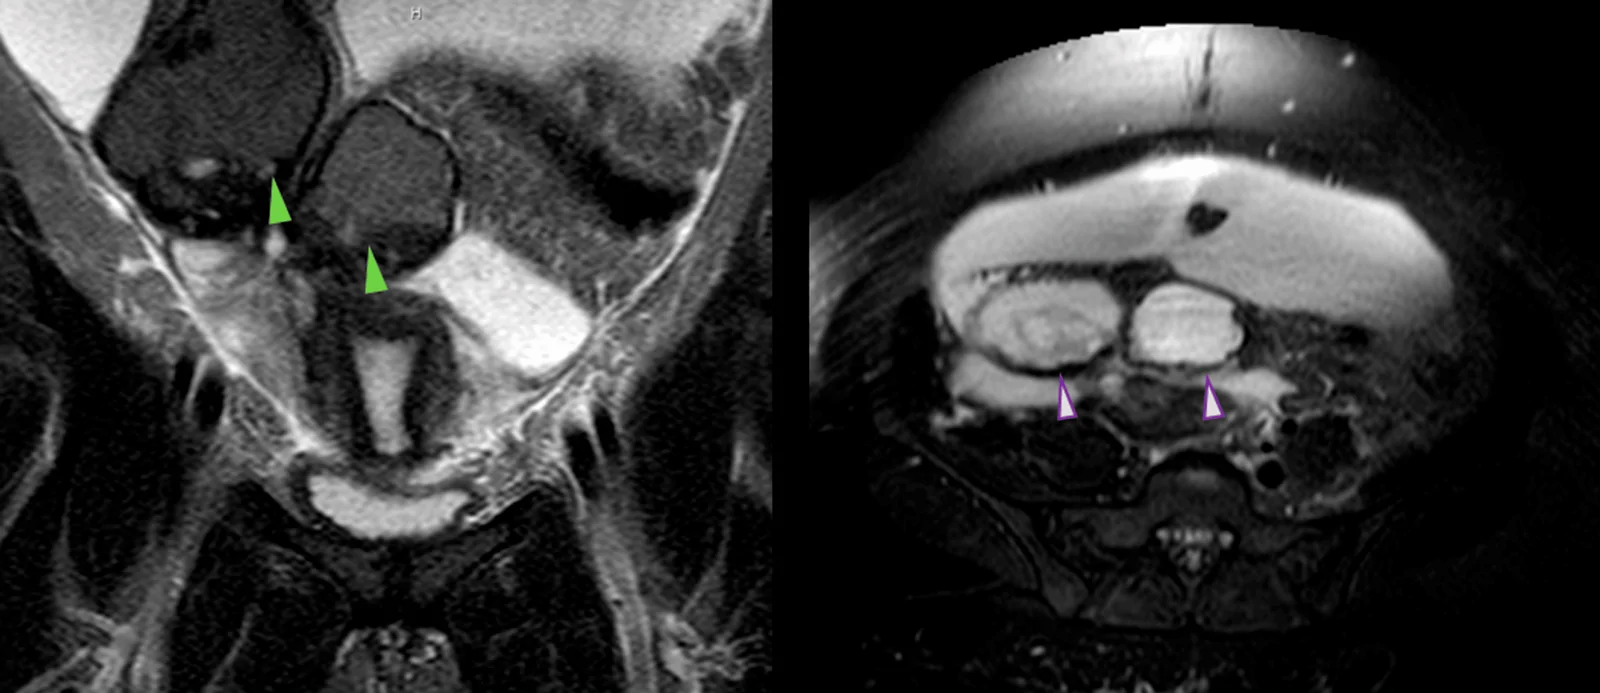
Pelvis MRI (coronal STIR and axial T2-Fatsat) The lesion shows a signal drop on inversion-recovery sequences (green arrowheads), a finding that can be mistakenly assumed to represent fat, but it remains high signal on T2 fat-saturated (T2-FS) images (pink arrowheads). The STIR sequence suppresses the signal from tissues with short T1 times, including methemoglobin, as in this case. MRI: magnetic resonance imaging; STIR: short tau inversion recovery
